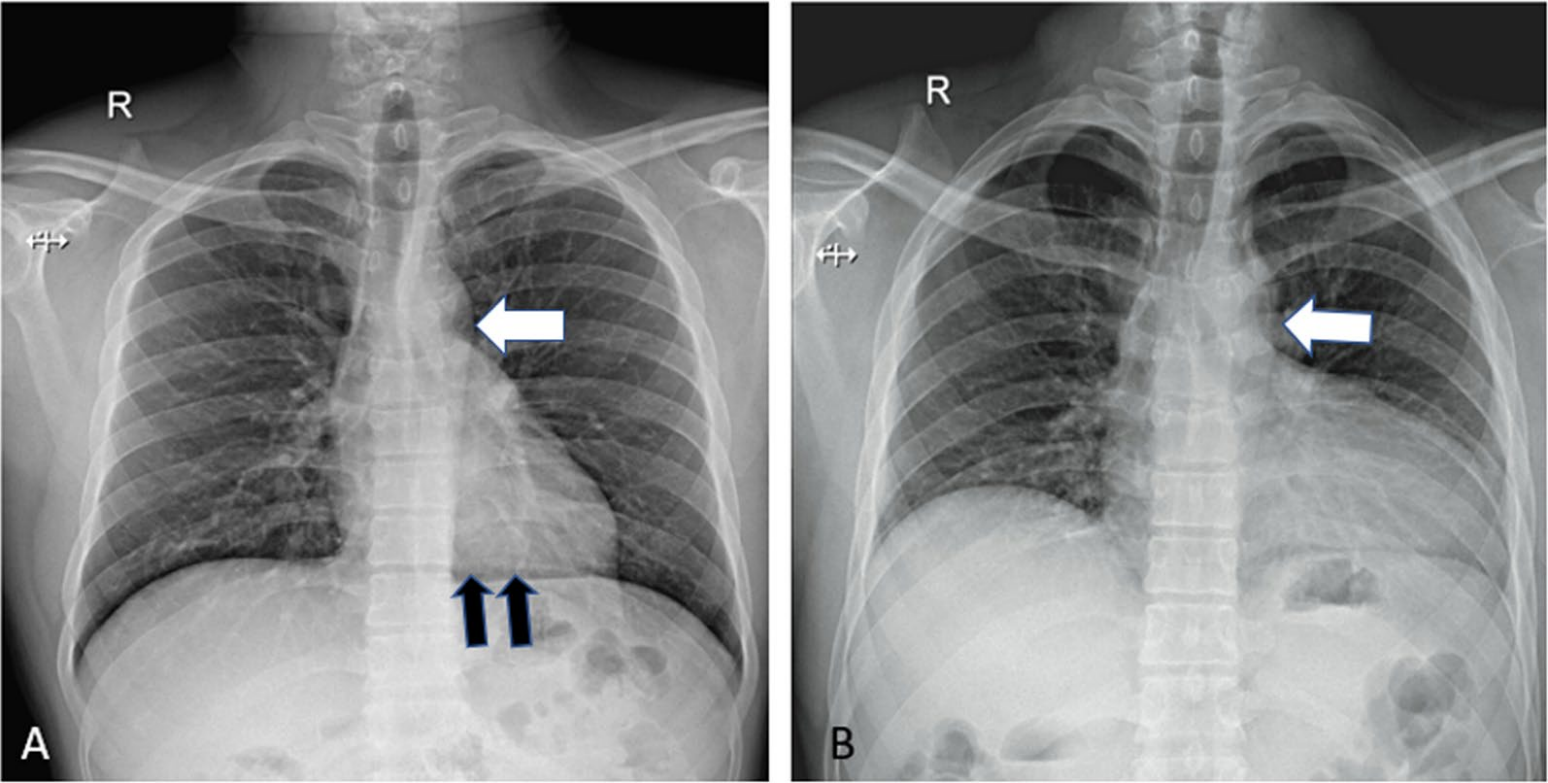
A Chest radiograph reveals normal position of left heart border and apex of heart with and a lucent area between the aorta and pulmonary artery (white arrow). B Chest radiograph (taken after 24 h) demonstrating the leftward position of the heart and the bulging contour of the left heart border lucent area between the aorta and pulmonary artery (white arrow). Lucency between the left hemidiaphragm and the base of the heart (black arrows)
